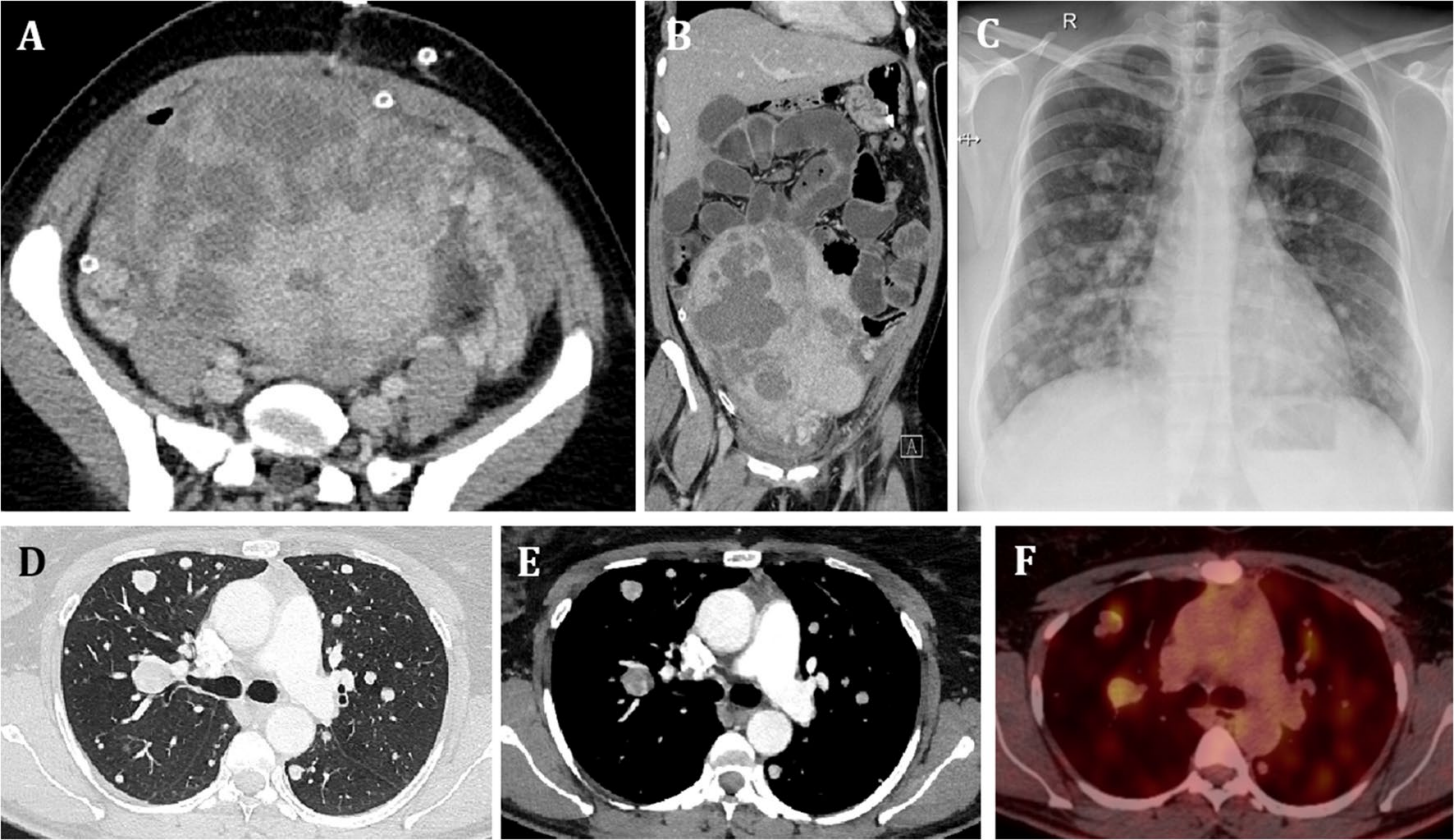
Imaging findings in Case 2. A, B Axial and coronal images from CT of the abdomen demonstrating the large heterogeneously enhancing leiomyomas. C Chest radiograph of the patient showing multiple radio-opaque nodular opacities with a variable size in both lungs. D Axial CT of the lung window showing multiple variable-sized randomly distributed lung nodules, typical of metastatic lung nodules. E Axial CT lung with a meditational window showing multiple nodules with heterogeneous densities and IV contrast enhancement. F Axial CT-PET fused images demonstrating high FDG uptake in the lung nodules
